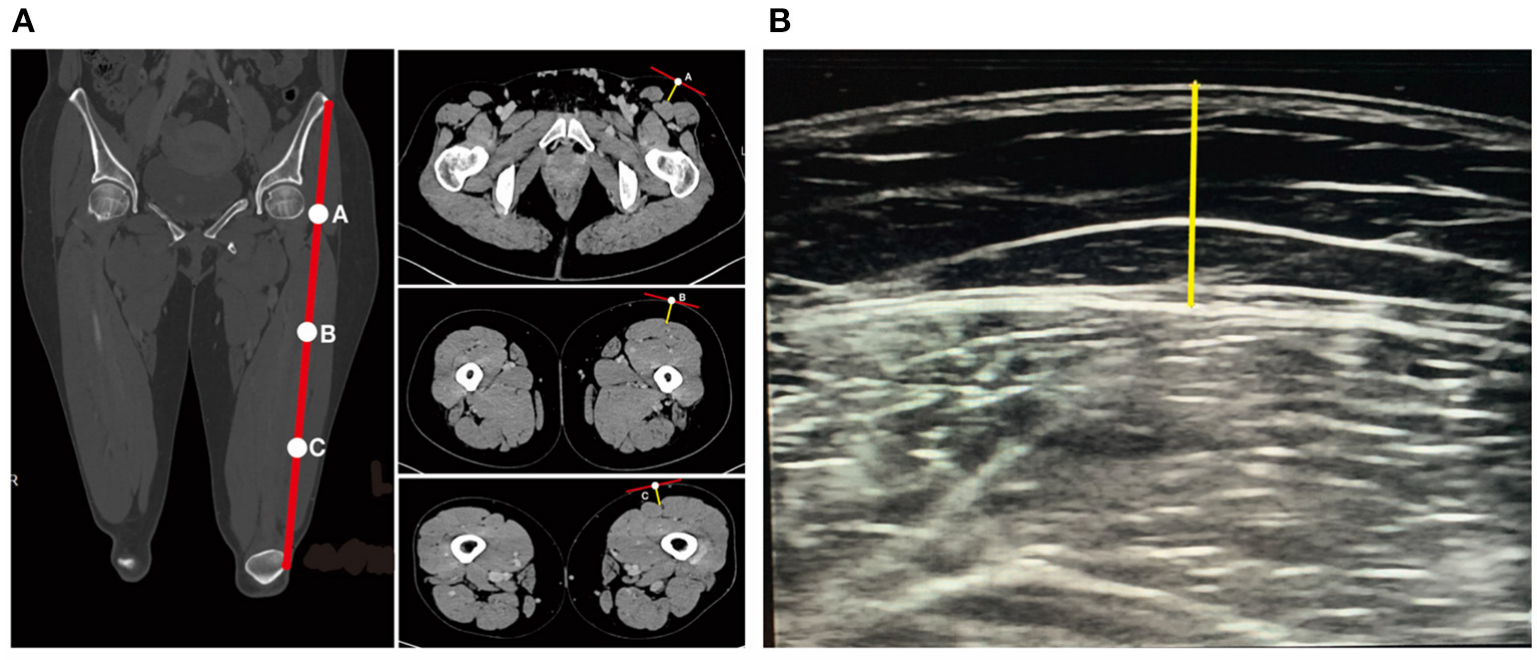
Measurements of ALT flap thickness by CT or ultrasound. (A) Measurement of ALT flap thickness by CT. Yellow straight line indicates the thickness of ALT flap. (B) Measurement of ALT flap thickness by ultrasound. Yellow straight line indicates the thickness of ALT flap.
